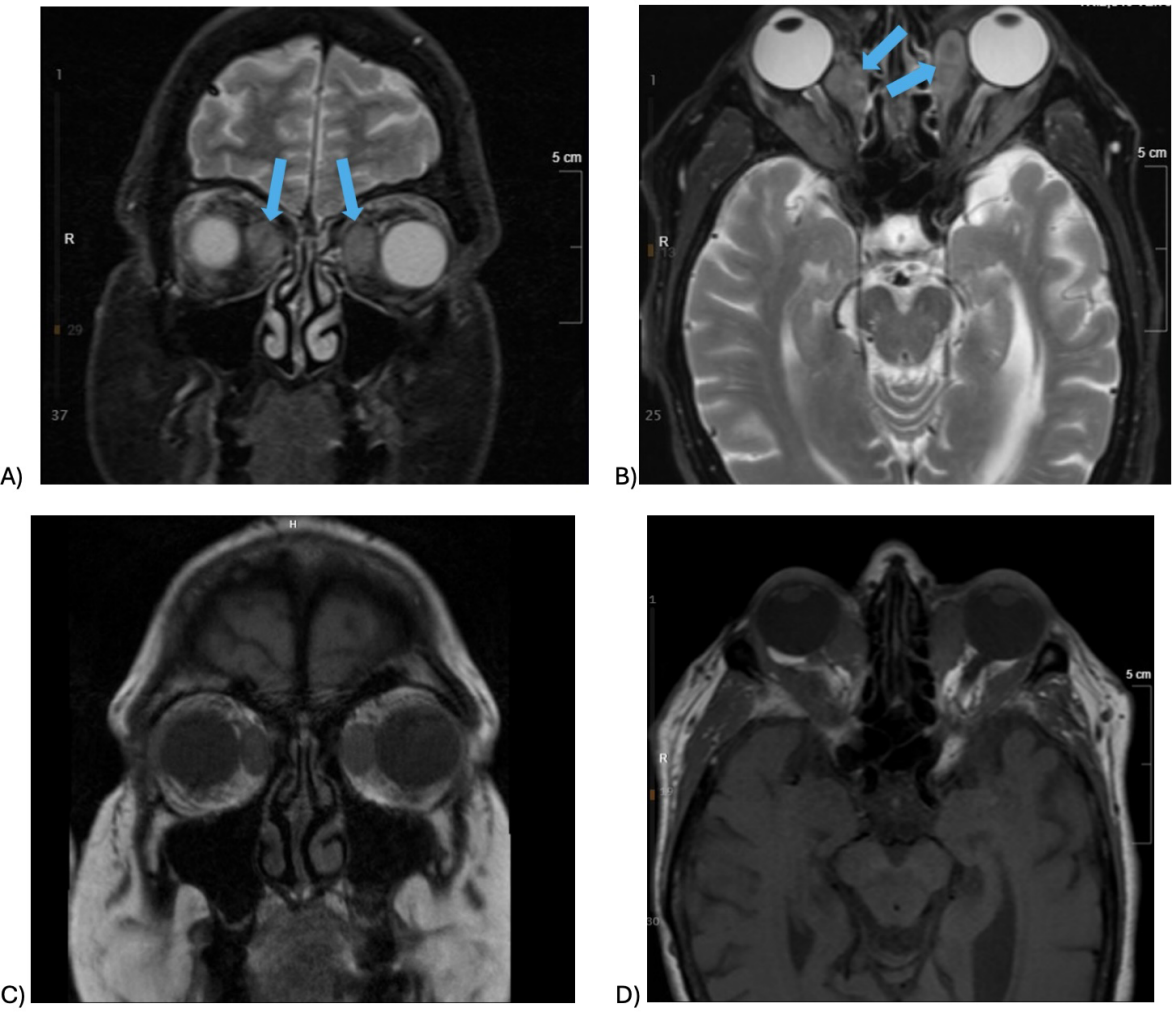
(A–D) MRI orbit without contrast performed in December 2022 showing bilateral perineuromas. (A) T2-weighted coronal view, (B) T2-weighted axial view, (C) T1-weighted coronal view, and (D) T1-weighted axial view.
